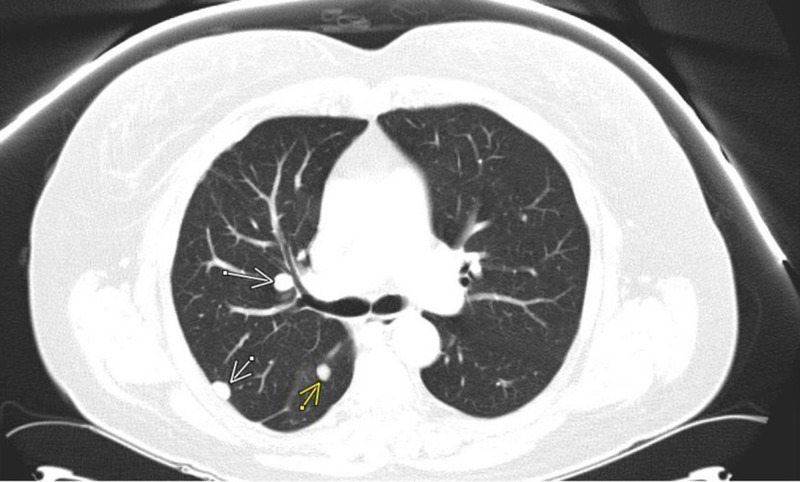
Chest CT scan 10/2015 showed pulmonary nodules increased in size.
